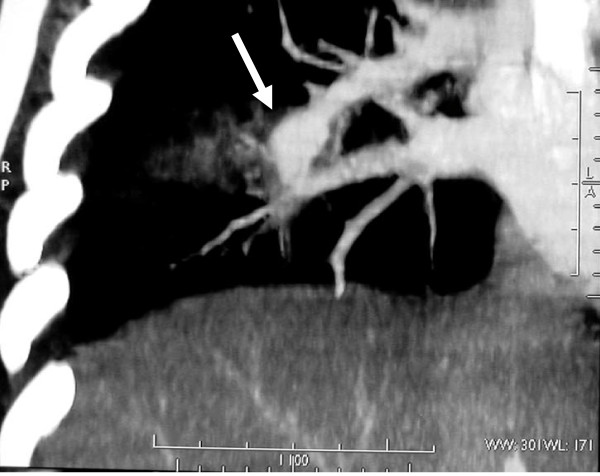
Cardiac magnetic resonance imaging showed the aneurysm in the right interlobar pulmonary artery (white arrow).
